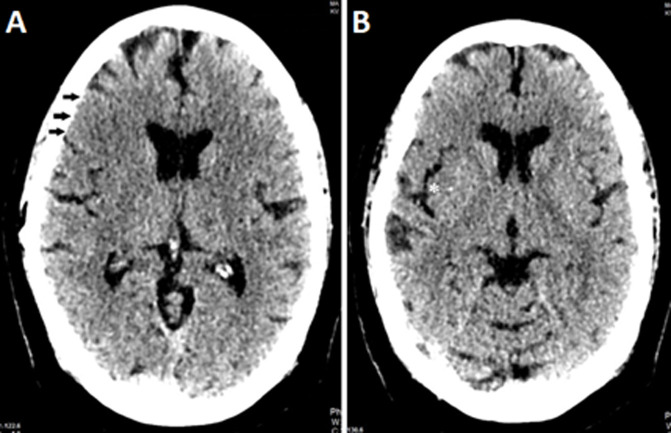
(A, B) brain computed tomography scan showed loss of grey-white matter differentiation (black arrows) and loss of right insular ribbon sign (*)
